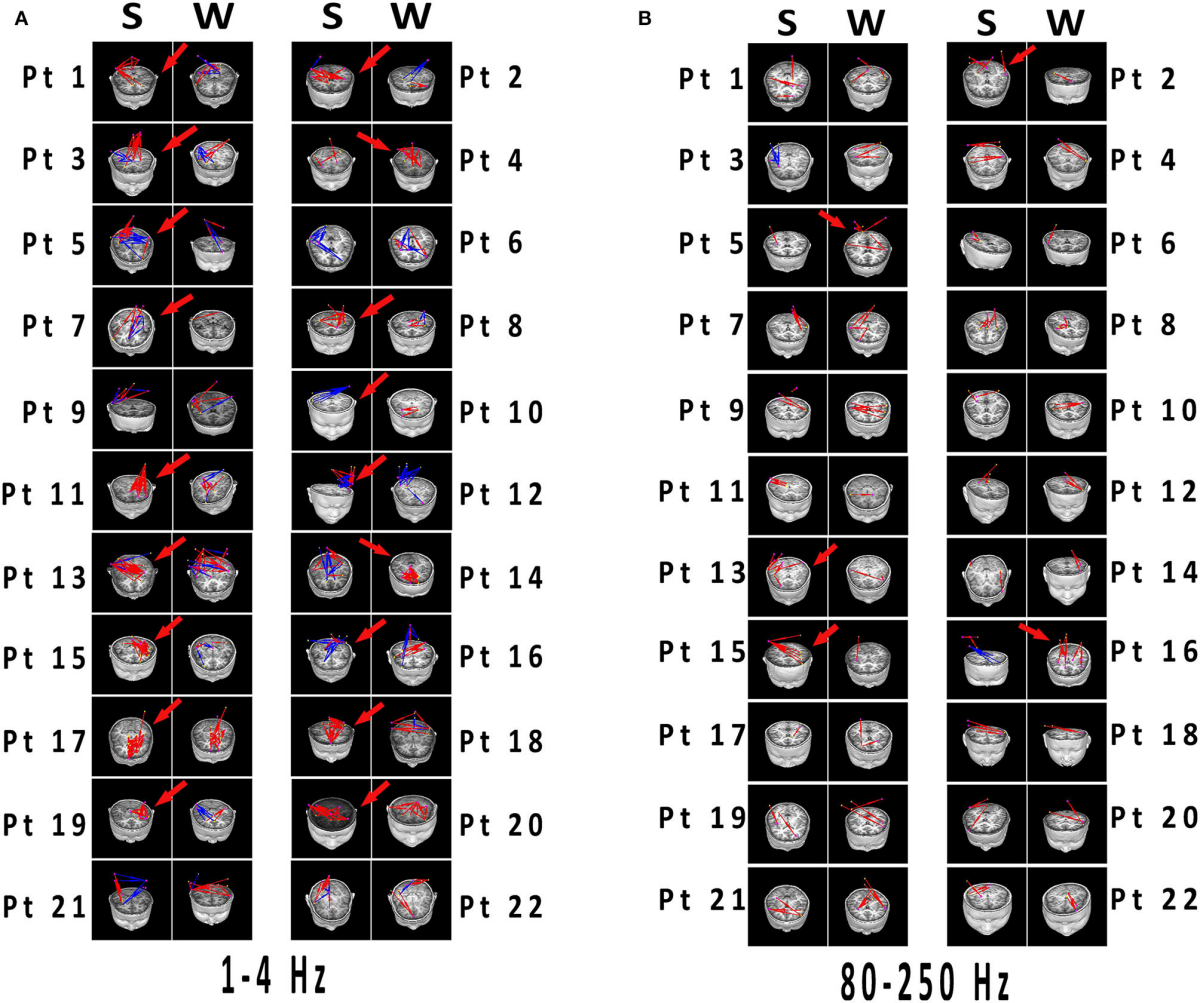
(A,B) Network connectivity distributions of spikes and slow waves registered to MRI. Significant differences have been labeled with red arrows. (A) 1–4 Hz was selected as the representation with typical distinctions, which the connections in spikes were more than that in slow waves with excitatory connections predominant. (B) At 80–250 Hz, both spikes and slow waves mostly showed excitatory connections. Differences were not significant in network distributions in most patients, and connections was less than that in lower frequency bands. Blue and red indicated inhibitory and excitatory connections, respectively. MRI, magnetic resonance imaging; S, spikes; W, slow waves; Pt, patient.
